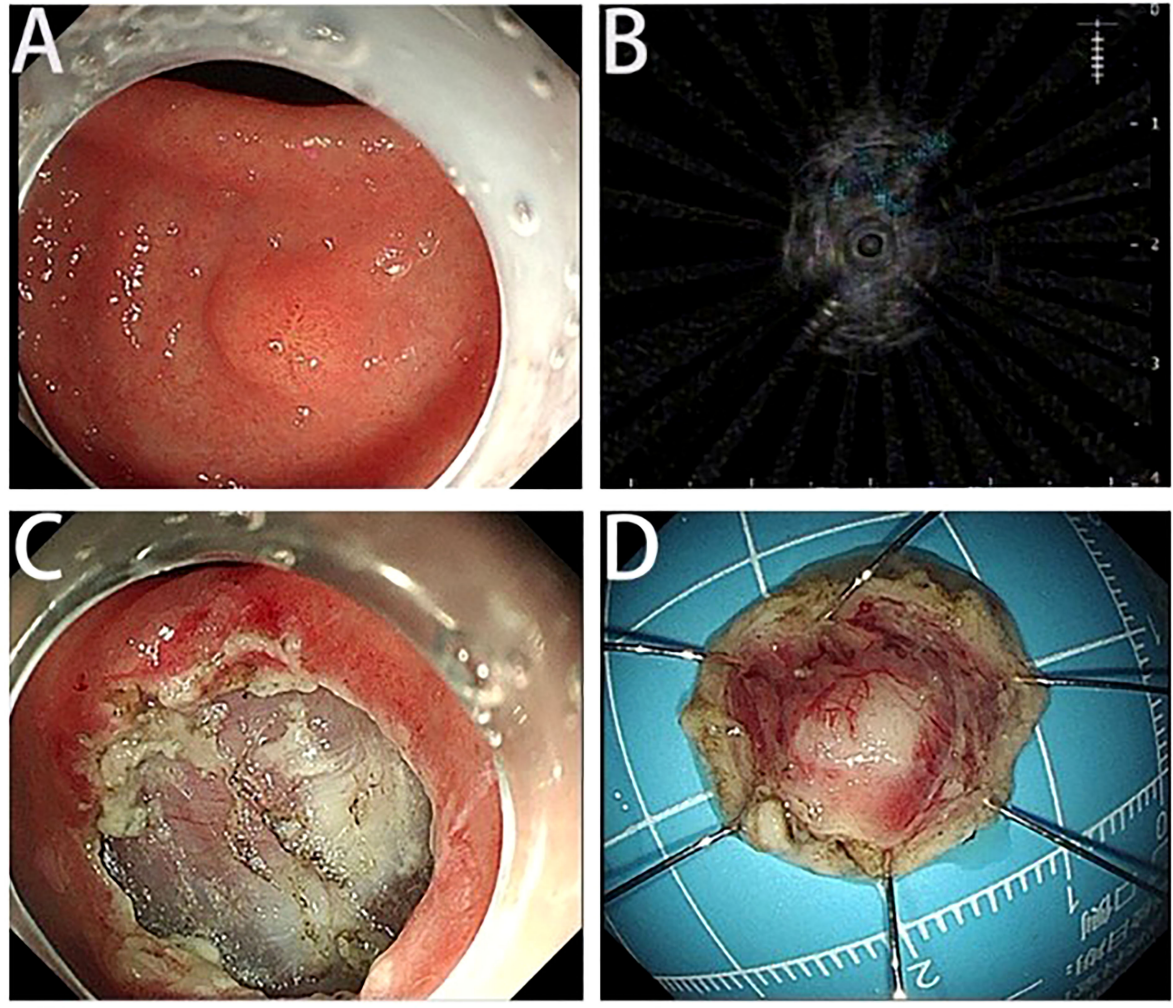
Endoscopic and endoscopic ultrasound findings and ESE images. (A) The upper gastrointestinal endoscopy shows a submucosal protrusion at the lesser curvature of gastric antrum. The tumor surface is covered by normal gastric mucosa. (B) The EUS shows the hypoechoic lesion originating from the submucosa. (C) The wounds after ESE. (D) The resected tumor tissue.
